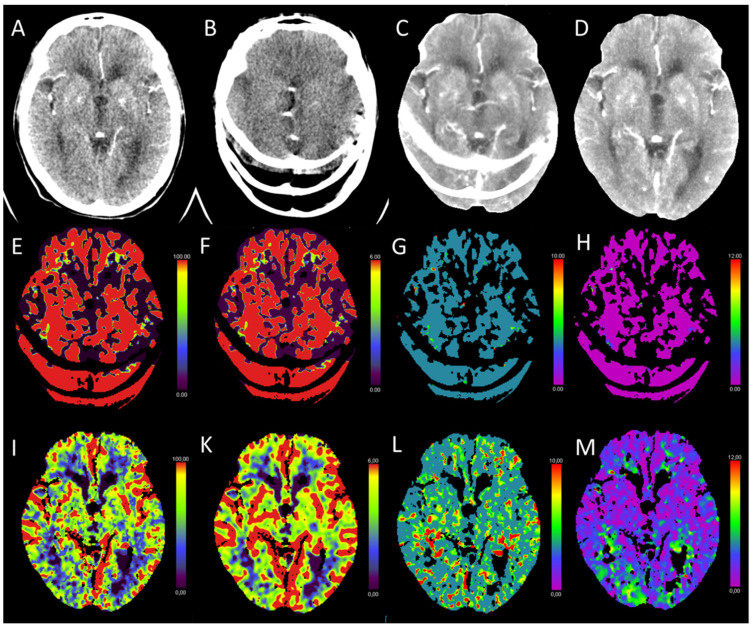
(A,B): computed tomography perfusion (CTP) of the brain at the same position at different time points. (C) maximum intensity projection (MIP) of the CTP generated by automated postprocessing software, (D) MIP of the CTP generated by manual postprocessing. (E–H): color-coded maps generated from CTP by automated postprocessing software: (E) cerebral blood flow (CBF) [mL/100 g/min], (F) cerebral blood volume (CBV) [mL/100 g], (G) mean transit time (MTT) [s], and H) time to maximum (Tmax) [s]. (I–M): color-coded maps generated from CTP by manual postprocessing: (I) CBF [mL/100 g/min], (K) CBV [mL/100 g], (L) MTT [s], and (M) Tmax [s]. Due to severe motion artifacts (B), the perfusion maps generated by automated postprocessing software algorithms were not usable for diagnostic purposes in this exemplary patient case. During the manual postprocessing steps, imaging time points with motion artifacts were excluded. The resulting manually generated perfusion maps (I–M) were usable and showed normal brain perfusion.
